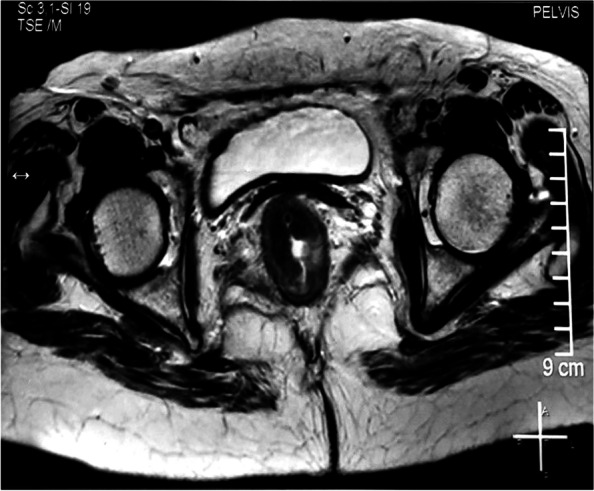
Axial view of MRI pelvis. Eccentric wall thickening (16 mm) involving distal rectum at right anterolateral aspect with heterogenous signal intensity, restricted diffusion and transmural extent, mesorectal fascia appears free, no significant locoregional lymph nodes
